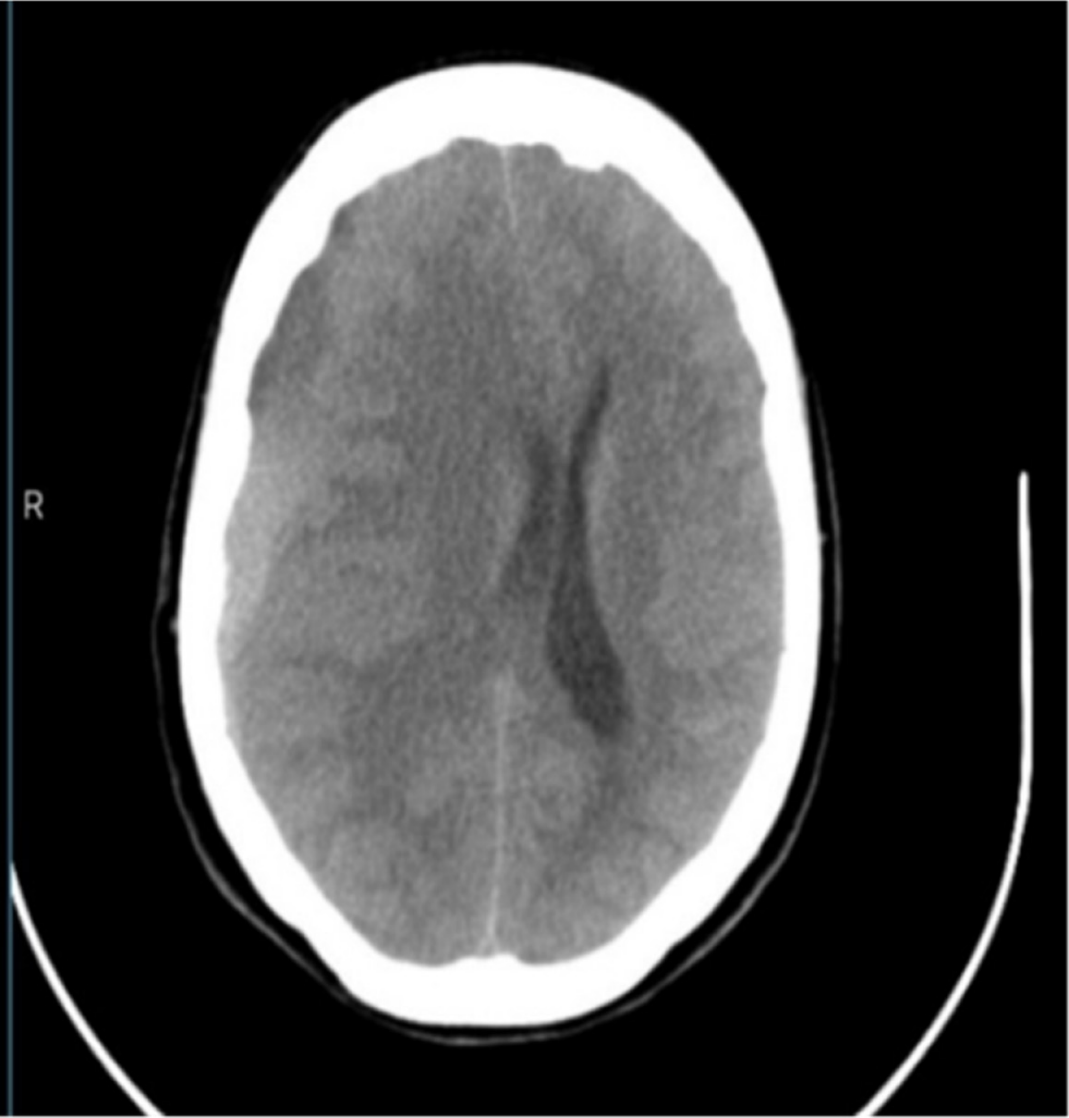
Computed tomography axial image showing acute on chronic right frontal parietal subdural hematoma measures 27 cm^3^ with midline shift of 1.7 cm with subfalcine herniation, ipsilateral trans tentorial, uncal hernation, and cerebellar tonsillar herniation by 5 mm. Positive for generalized cerebral edema and mild left obstructive ventriculomegaly.
